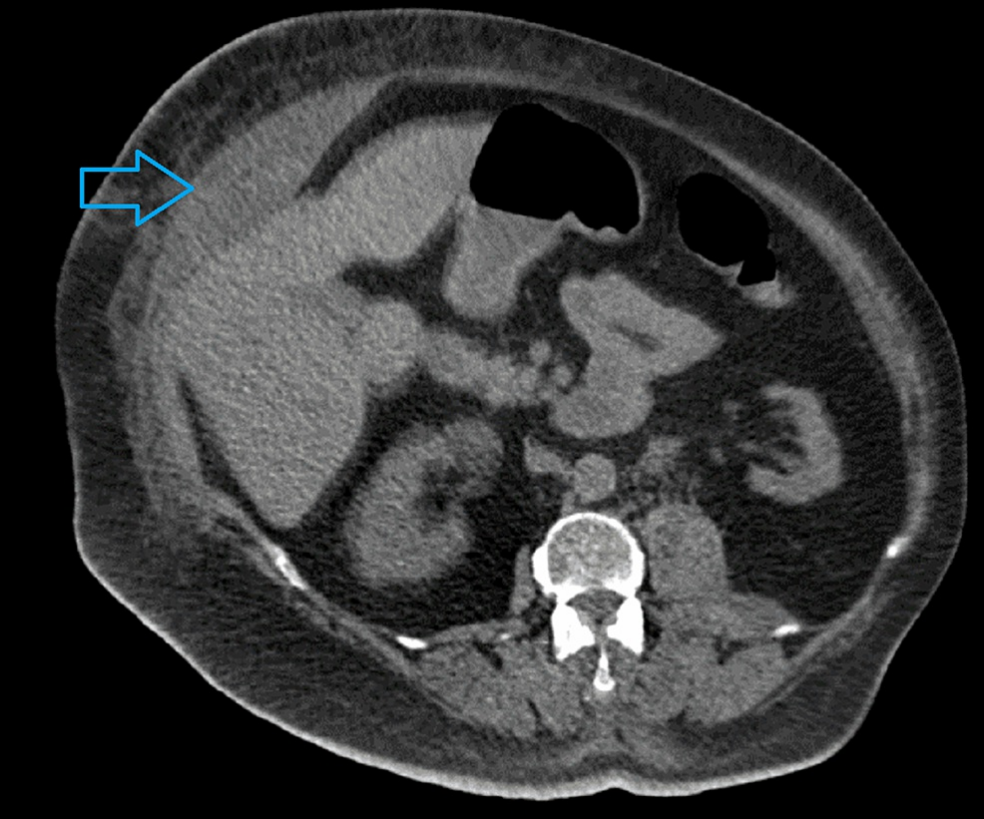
Right internal oblique hematoma (arrow) on the same CT scan.
